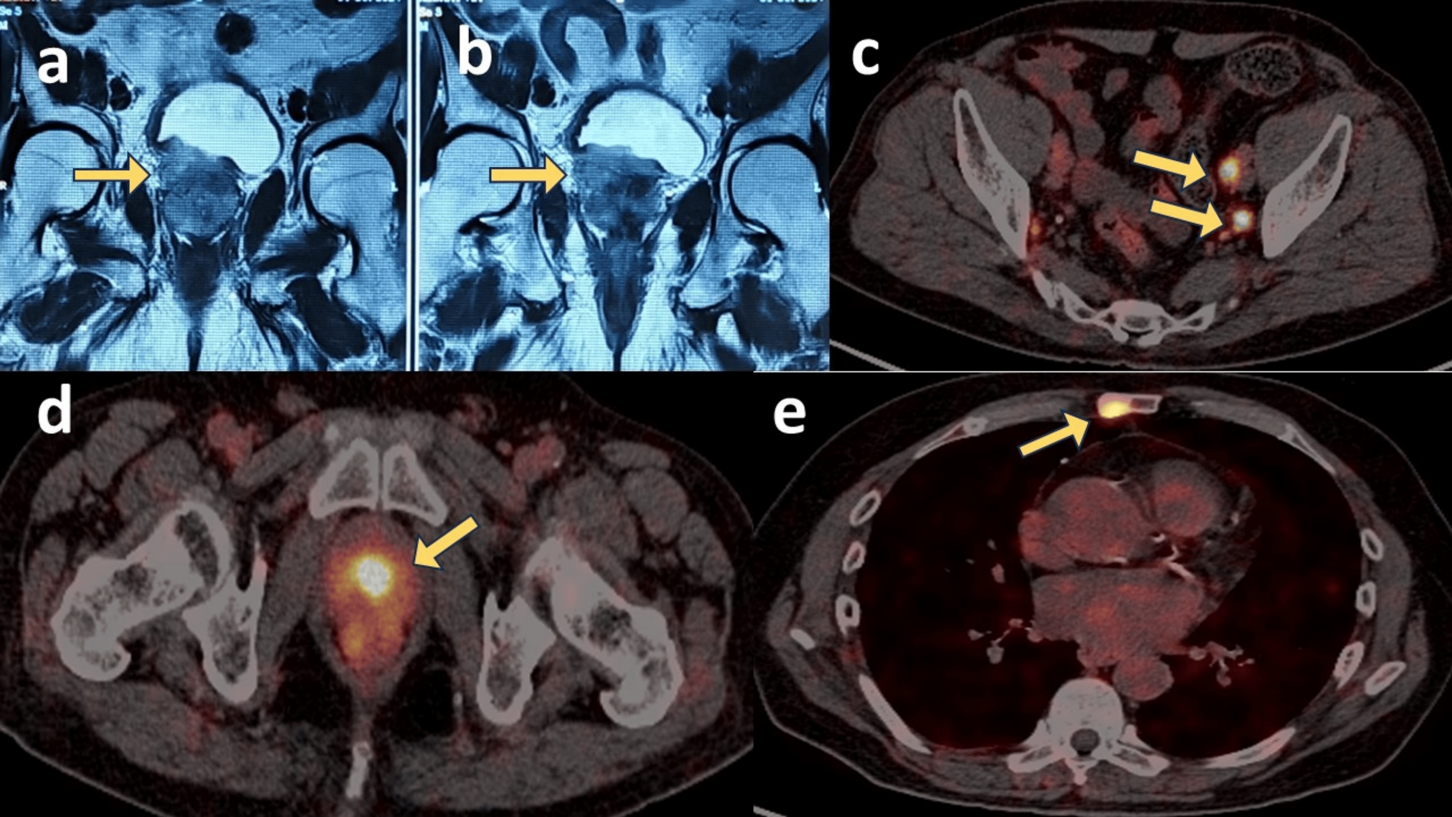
MpMRI and PSMA PET-CT appearances of the prostatic primary and metastatic sites a: There was diffuse nodularity of the central and transitional zones of the prostate (88 cc in volume) with heterogeneous parenchyma. b: The right-side base of the peripheral zone was moderately bulky and showed a well-defined, nodular lesion in the median and para-medial locations. The lesion measured approximately 3.7 x 3 cm, was isointense on T1W (T1-weighted) images, hypointense on T2W (T2-weighted) sequences, with restricted diffusion, hyperintense on DWI (diffusion-weighted imaging), and darkening on ADC (apparent diffusion coefficient). The lesion showed intense enhancement on T1WFS (T1-weighted fat-saturated) post-gadolinium images. The prostatic capsule was breached, and neurovascular bundles were infiltrated. The right seminal vesicle and levator ani were bulky, with loss of planes suggestive of infiltration. There was a contiguous infiltration of the bladder base and right lateral wall. c: Multiple PSMA-avid pelvic lymph nodes on PSMA PET-CT scan. d: Intense PSMA-avidity of the prostatic primary malignancy. e: PSMA-avid sternal lesion (metastatic site). MpMRI: multiparametric magnetic resonance imaging; PSMA PET-CT: prostate-specific membrane antigen positron-emission tomography-computed tomography
